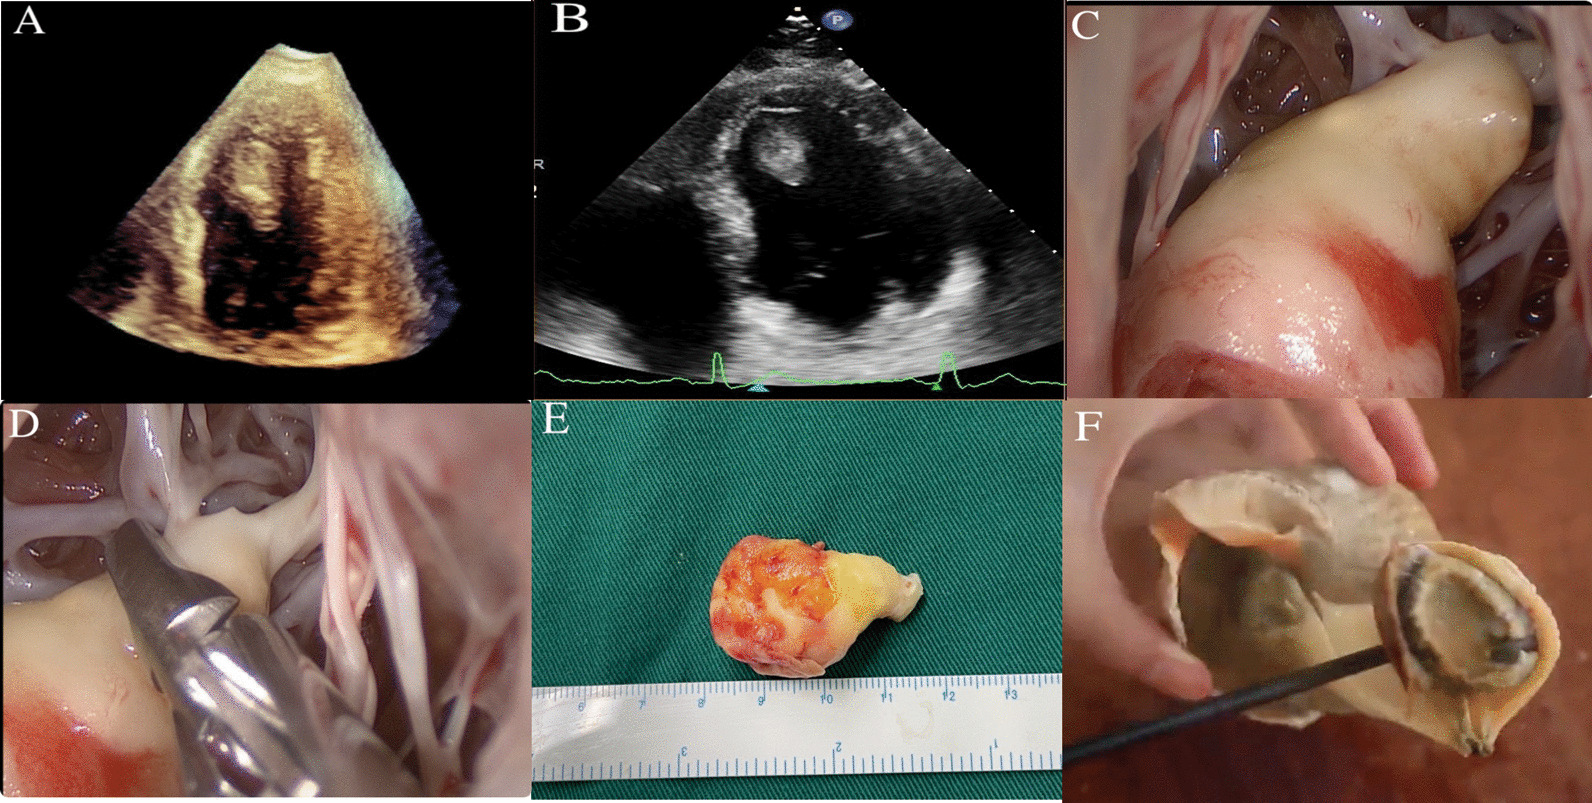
A, B Transthoracic echocardiography revealed that a spiral cystic mass, 27 × 14 mm, located on the left ventricular apex. It looked like a conch floating in the left ventricle. C, D Intraoperative findings: A spiral cystic shape and 1.5 * 5 cm size of mass rooted on the left ventricular apex. The tumor was mainly soft and fragile, while the basement was partly calcified. E, F The conch has a similar silhouette and is an appropriate eponym of this sign
